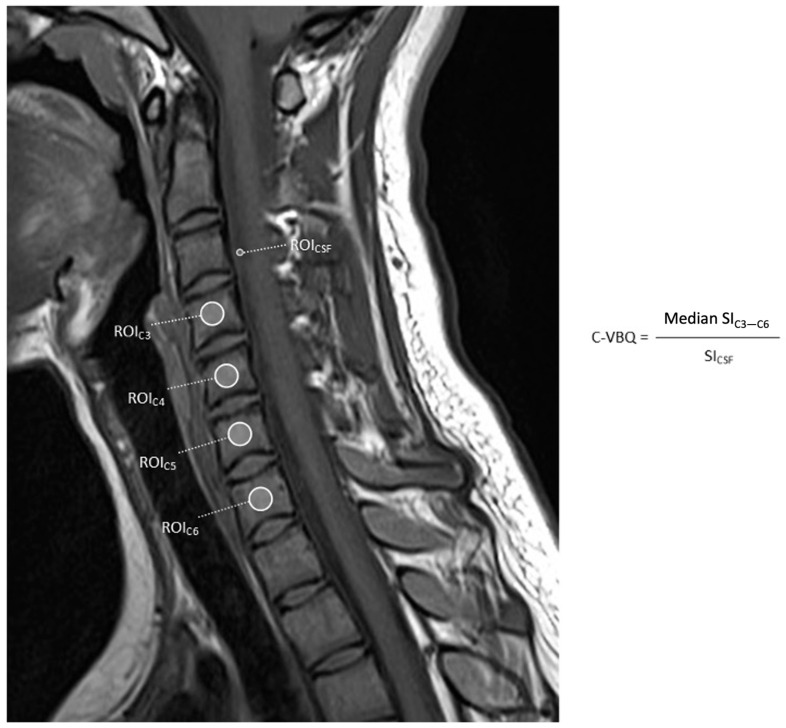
Non-contrast-enhanced T1-weighted MRI of the cervical spine showing ROIs used to calculate C-VBQ.
